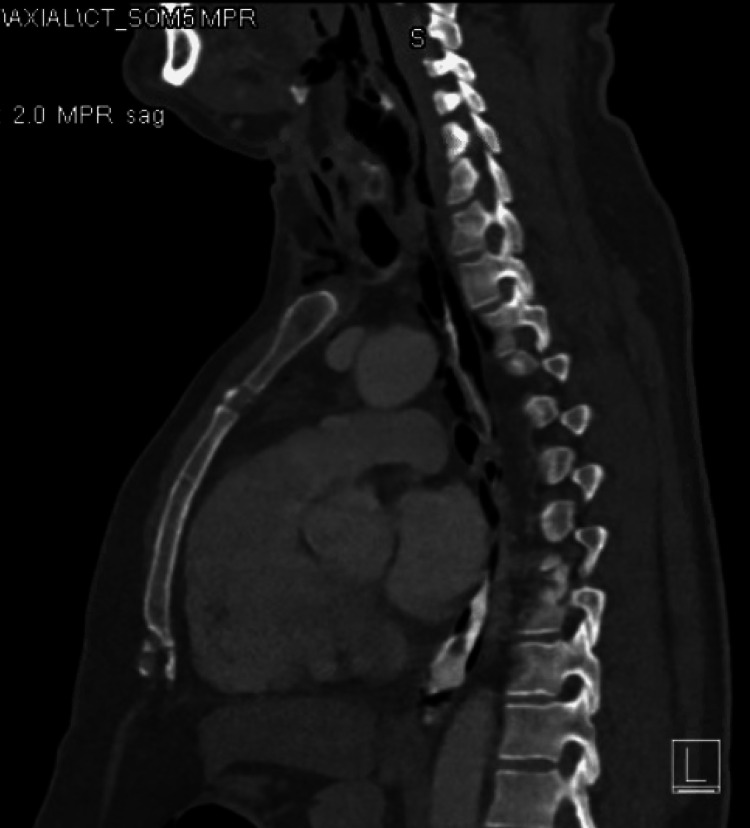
Sagittal CT imaging of the neck and chest.
